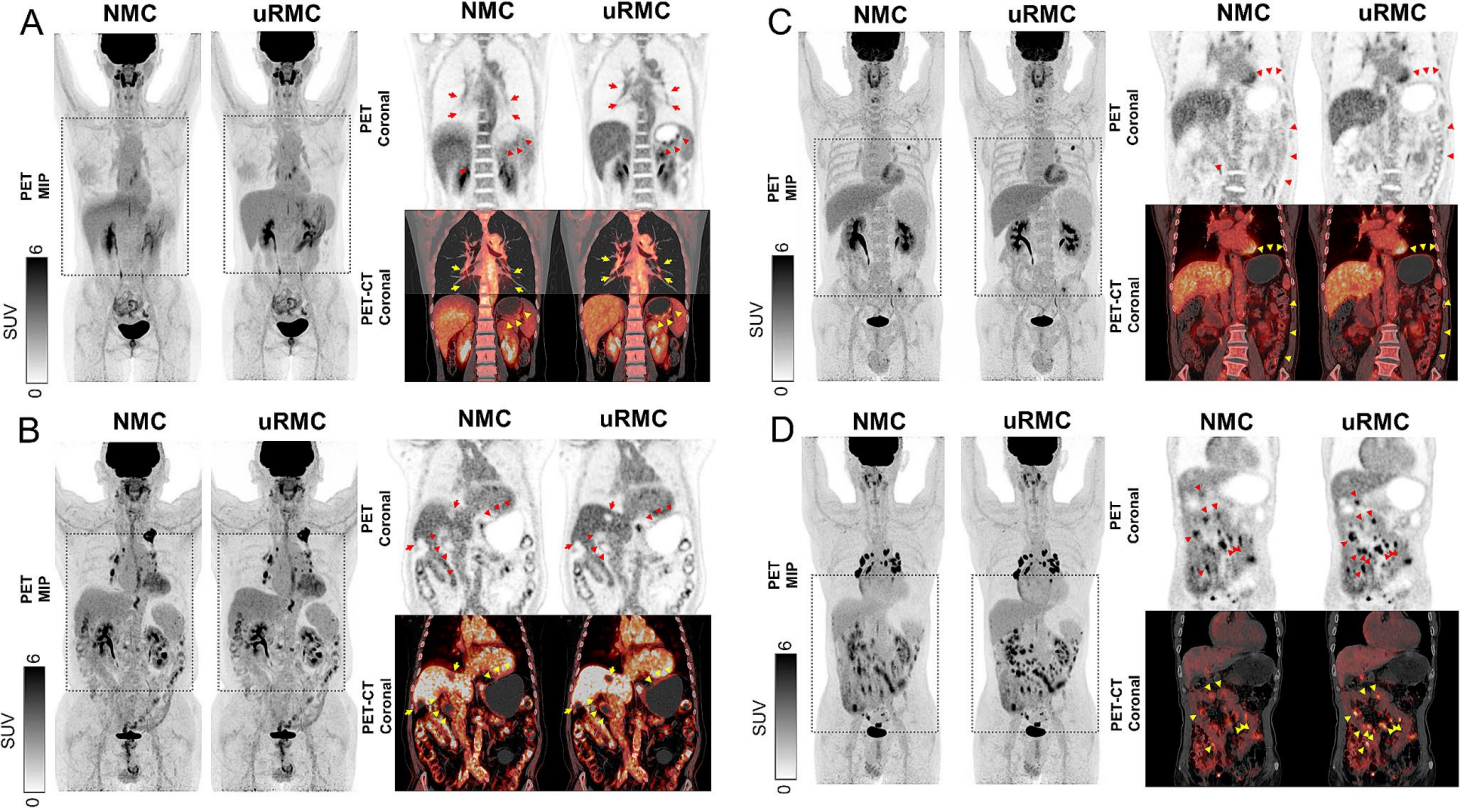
Demonstrating PET delineation and PET-CT alignment differences between NMC and uRMC images for physiological and/or benign uptakes. (A) Bronchovascular tree, gastric folds, and hepatosplenic margin are better delineated in uRMC images comparing to blurred NMC images. (B) uRMC images reveal cold regions of liver cysts, uptakes of diaphragm, gastric linings, lower esophageal sphincter. (C) Gastric folds and the intestinal lining of the descending colon. (D) Mesenteric lymph nodes exhibit improved PET recovery and alignment with CT in uRMC images compared to NMC images. NMC: no motion correction; uRMC: unified respiratory motion correction
